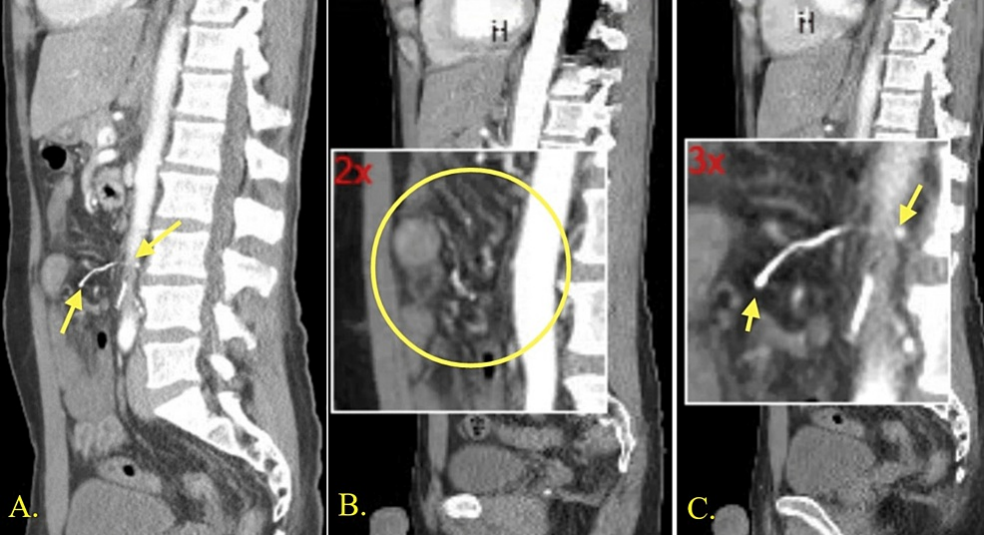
Sagittal views of CT images Images 1A-1C show an IVC filter wire strut measuring 25 mm penetrating the inferior vena cava into the peritoneal cavity CT, computed tomography; IVC, inferior vena cava
